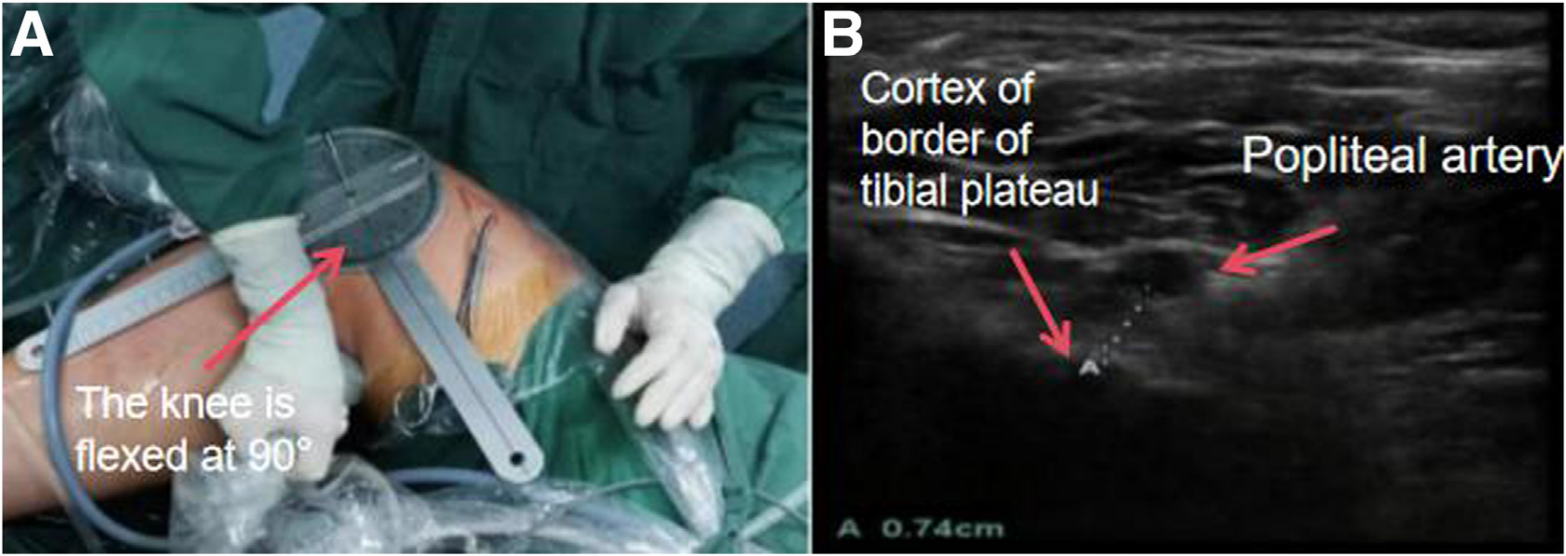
(A) The patient’s knee flexed at 90°. (B) Ultrasound probe is used to measure the distance on the horizontal axis (side, right; position “4”).
